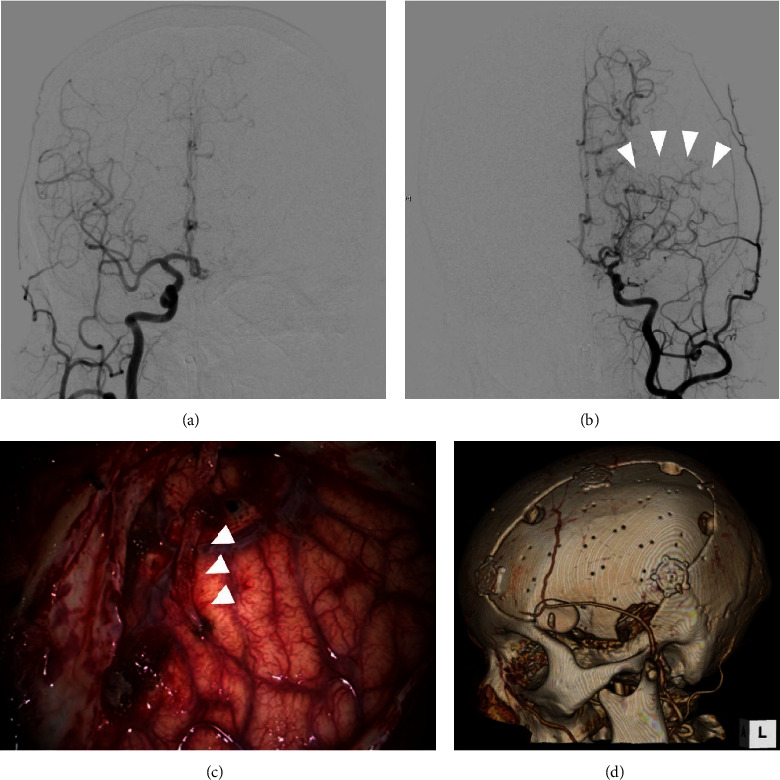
Images after initial procedure. (a) Digital subtraction angiography (DSA) of the right common carotid artery (CCA) after the initial surgery showing no remarkable abnormality. (b) DSA of the left CCA showed stenosis of the middle cerebral artery and moyamoya vessels (arrowheads). (c) Intraoperative photograph demonstrating encephalo-arterio-synangiosis between the brain surface and the parietal branch of the superficial temporal artery (STA) (arrowheads). (d) Three-dimensional computed tomography after the second surgery showing the structure of the implanted bone flap and STA.
